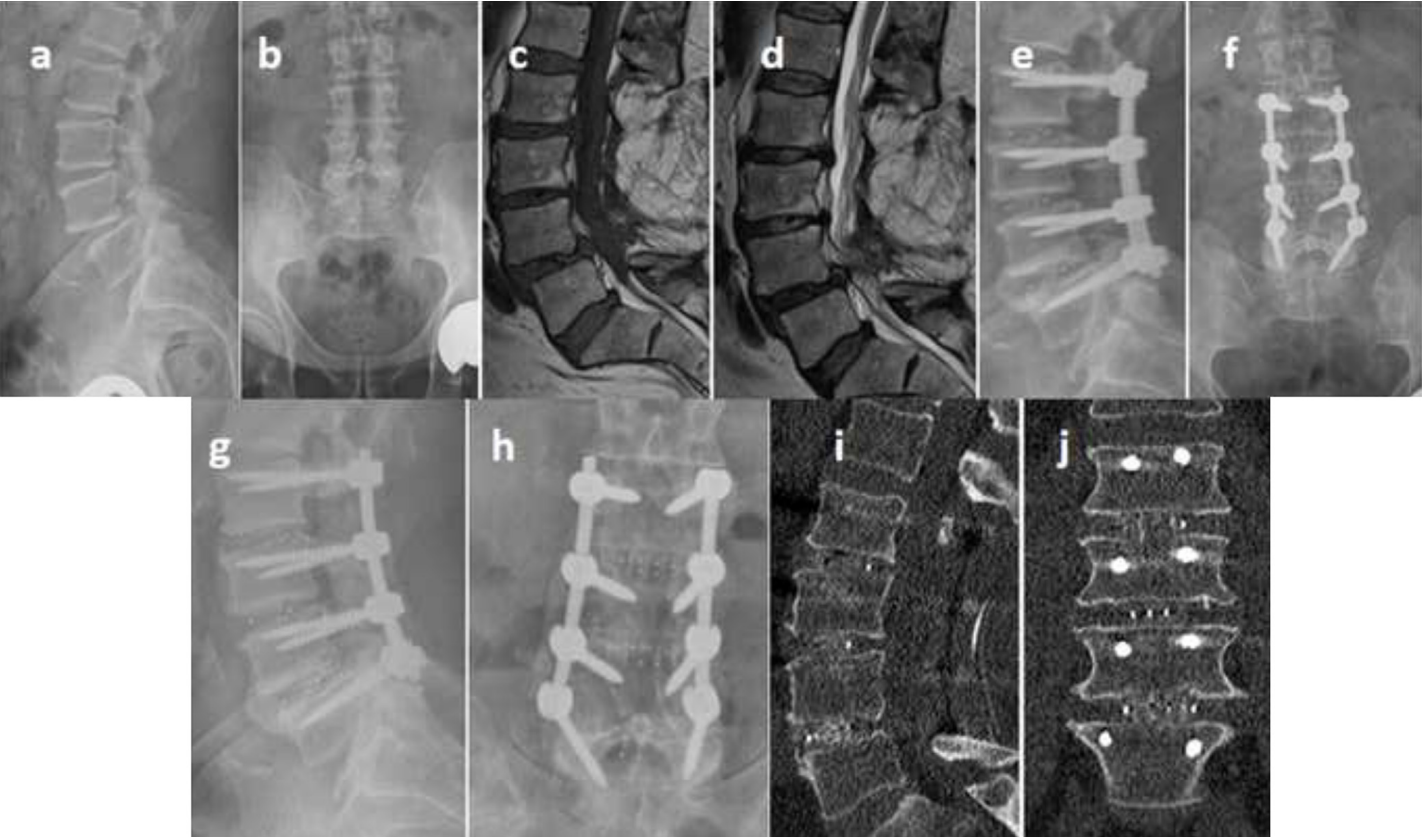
(a–d) Preoperative radiographs and MRI of 68-yrs male with postlaminectomy instability treated with TLIF L2:L5 using modular cages, (e,f) postoperative radiographs, (g–j) bony fusion was achieved at 1 year follow-up.
